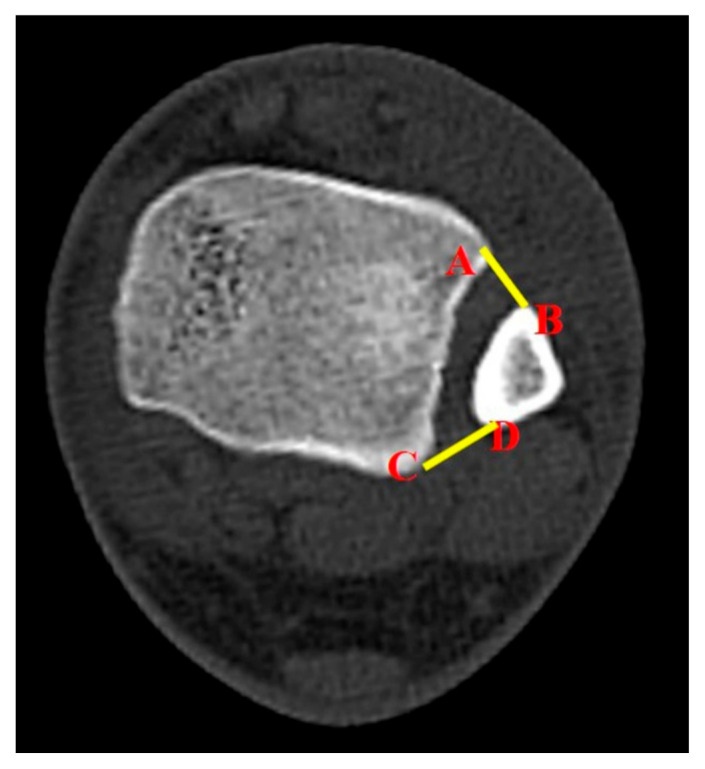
The surface area of syndesmosis, which was measured as a new parameter between four anatomic landmark points on the axial CT image; the points that link the anterior colliculus of the tibia (A), the most anterior aspect of the fibular (B), the posterior colliculus of the tibia (C), and the most posterior aspect of the fibular (D).
